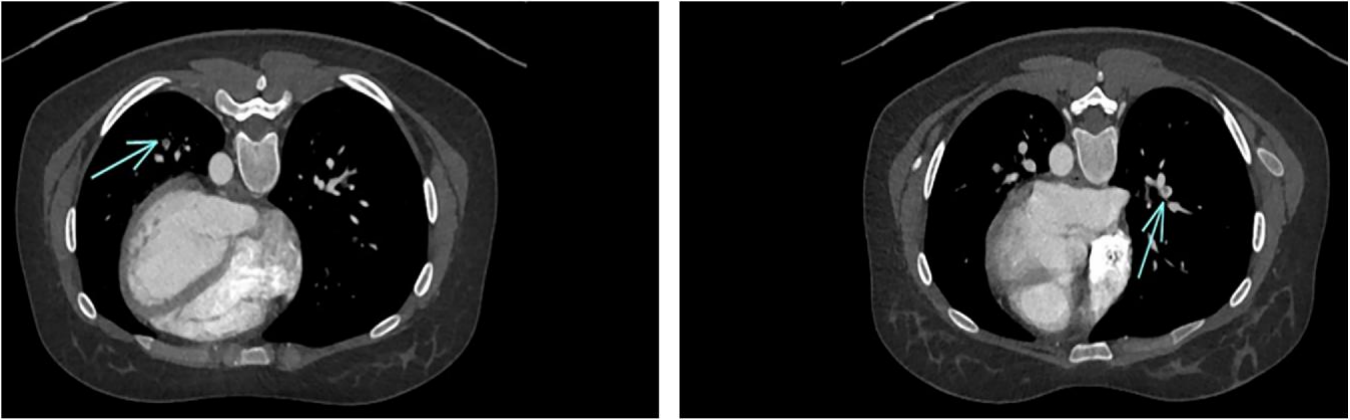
Thoraco-abdominopelvic CT scan at Day 20 revealed an endoluminal defect of the right inferior lobal artery, latero-basal segmentary, and left posture-basal artery, an image of webs seen at the right side, and absence of proximal pulmonary embolism (see arrows).
